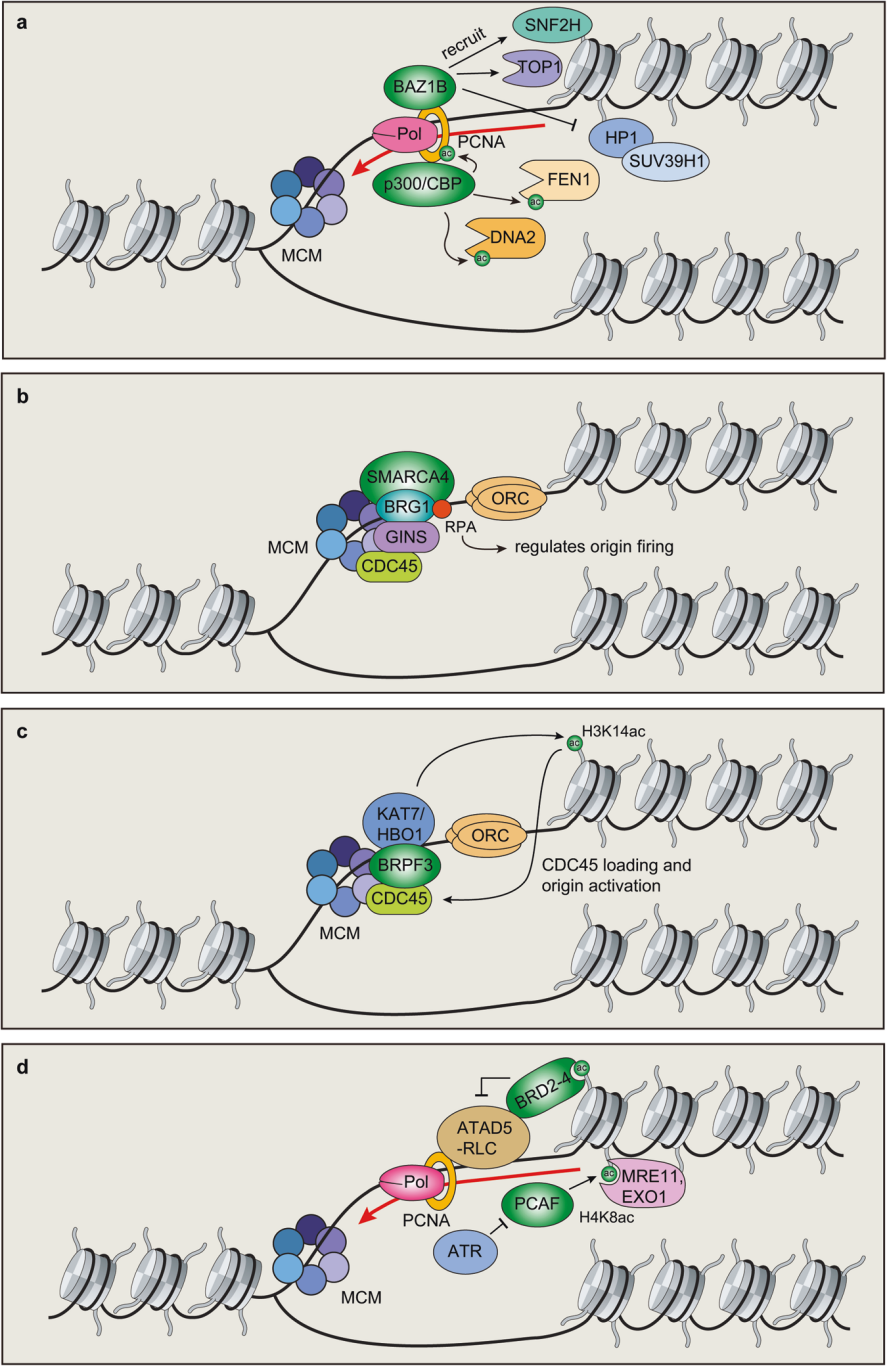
Bromodomain (BRD) proteins function in replication stress responses. a BAZ1B recruits SNF2H and topoisomerase I (TOP1) to replication forks, which inhibits HP1/SUV39H1-mediated H3 methylation and heterochromatin. p300/CBP acetylates PCNA, FEN1, and DNA2, which promote genome stability during replication. b SMARCA4/BRG1 regulates origin firing to protect genome stability. c BRPF3/HBO1 regulates origin activation by promoting H3K14ac and CDC45 loading. d BET proteins regulate PCNA levels at replication forks by regulating ATAD5 activity. PCAF acetylates H4K8 to recruit MRE11/EXO1 to stalled replication forks in BRCA-deficient cells. PCAF activity at stalled forks is limited by ATR phosphorylation.
